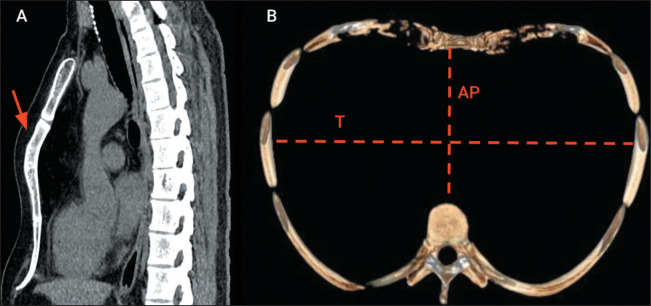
Pectus carinatum. A: Sagittal CT scan, with soft tissue window
